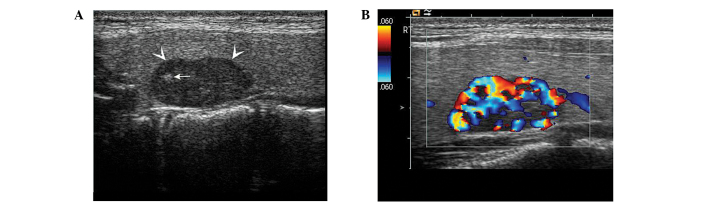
Color Doppler ultrasound demonstrating (A) non-uniform hypoechoic signals and an indication of calcified spots (white arrows). (B) Color Doppler flow imaging indicted increased blood flow signals.
